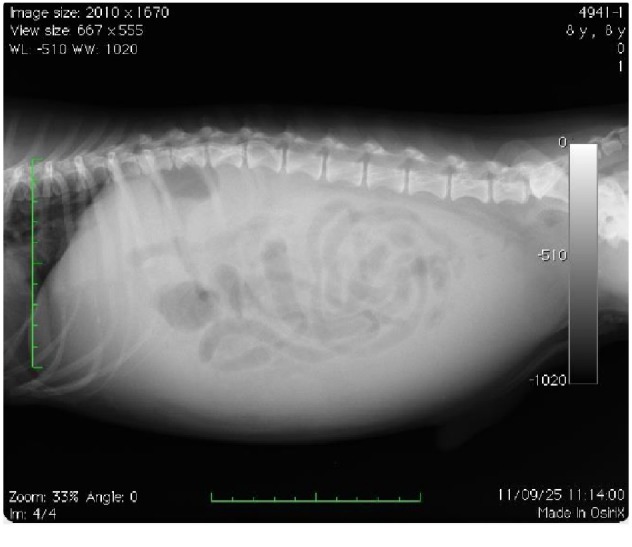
Abdominal radiograph showing ascites.
